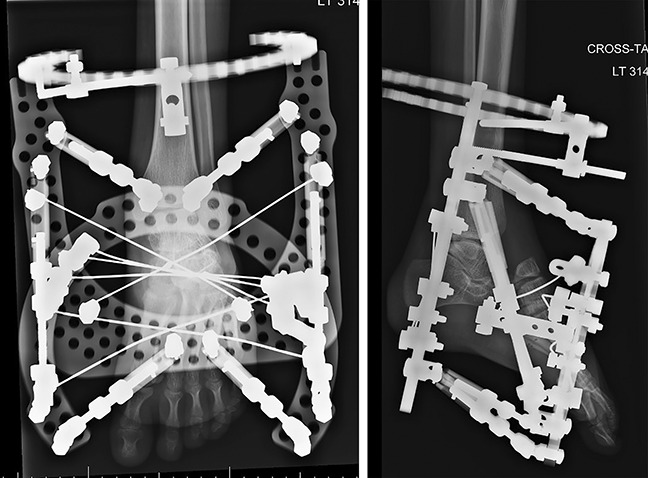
Anteroposterior and lateral postoperative radiographs (early stage 1) demonstrating the application of the hexapod fixator.
